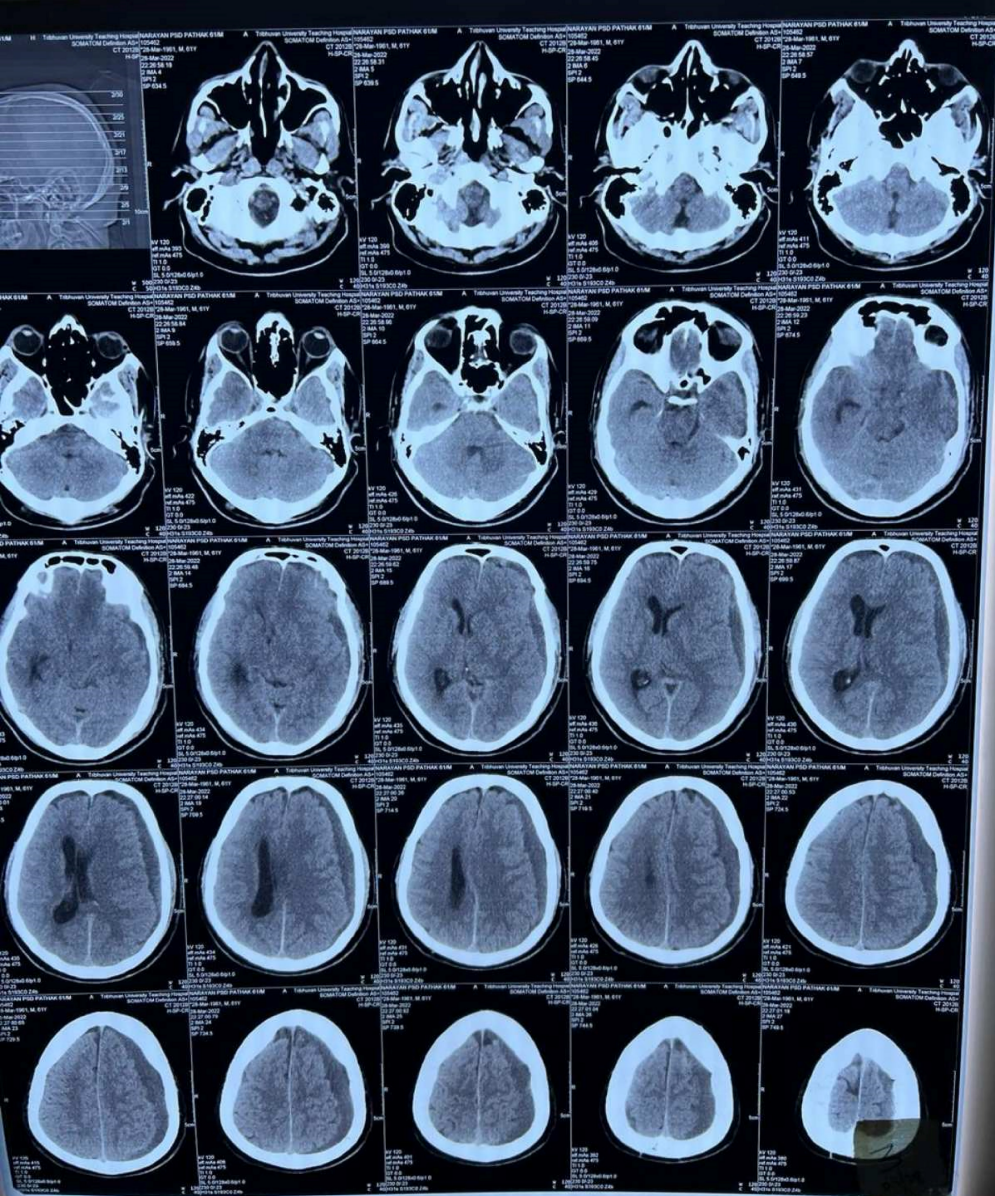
CT scan showing accumulation of blood in the subdural space with midline shift during the initial presentation.
